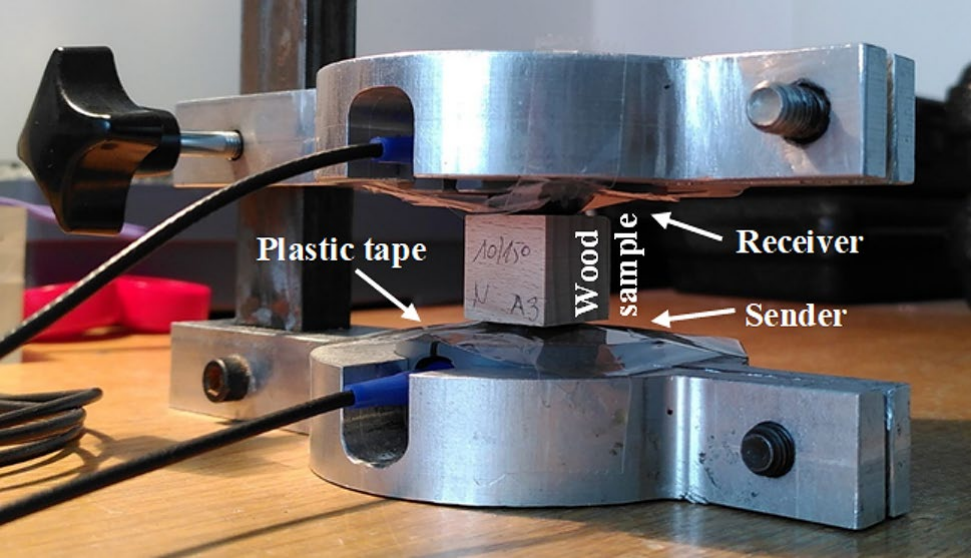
Ultrasound experimental setup.
